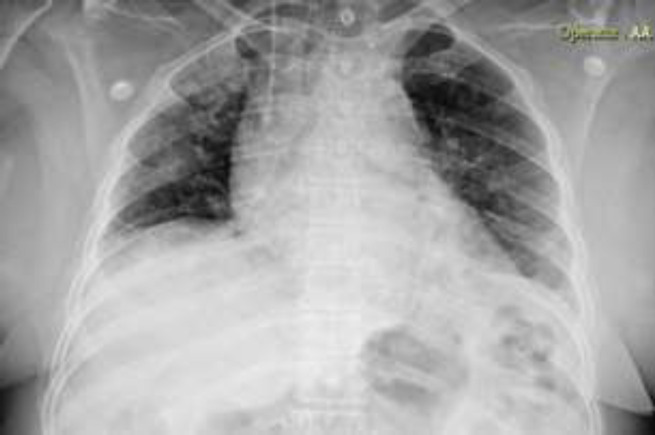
The lung infiltration resolved in the chest X-ray following the treatment
